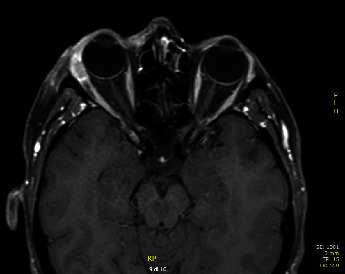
Magnetic resonance imaging showing local relapse in the form of multiple millimetric spread inside the superior orbital muscle.
